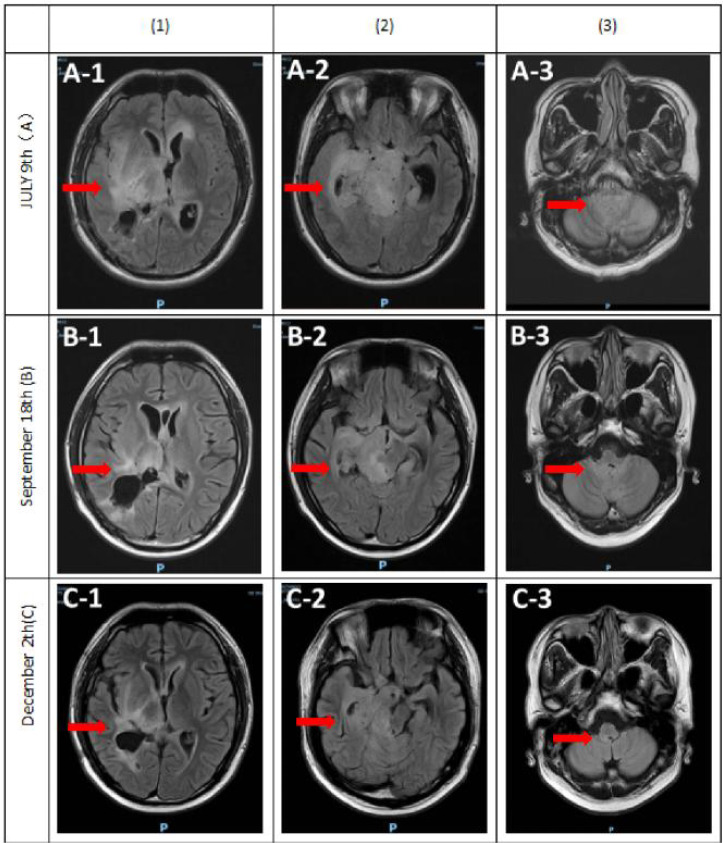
Brain MRI (T2 FLAIR Images). (A1-A3)Postoperative MRI following subtotal tumor resection. (B1-B3) Follow-up MRI obtained over two months (September 18) after concurrent chemoradiotherapy and targeted therapy, showing mild reduction in lesion size. (C1-C3) Follow-up MRI (December 2) after five cycles of combined teniposide, temozolomide, and bevacizumab therapy, demonstrating marked tumor regression and decreased midline shift.
